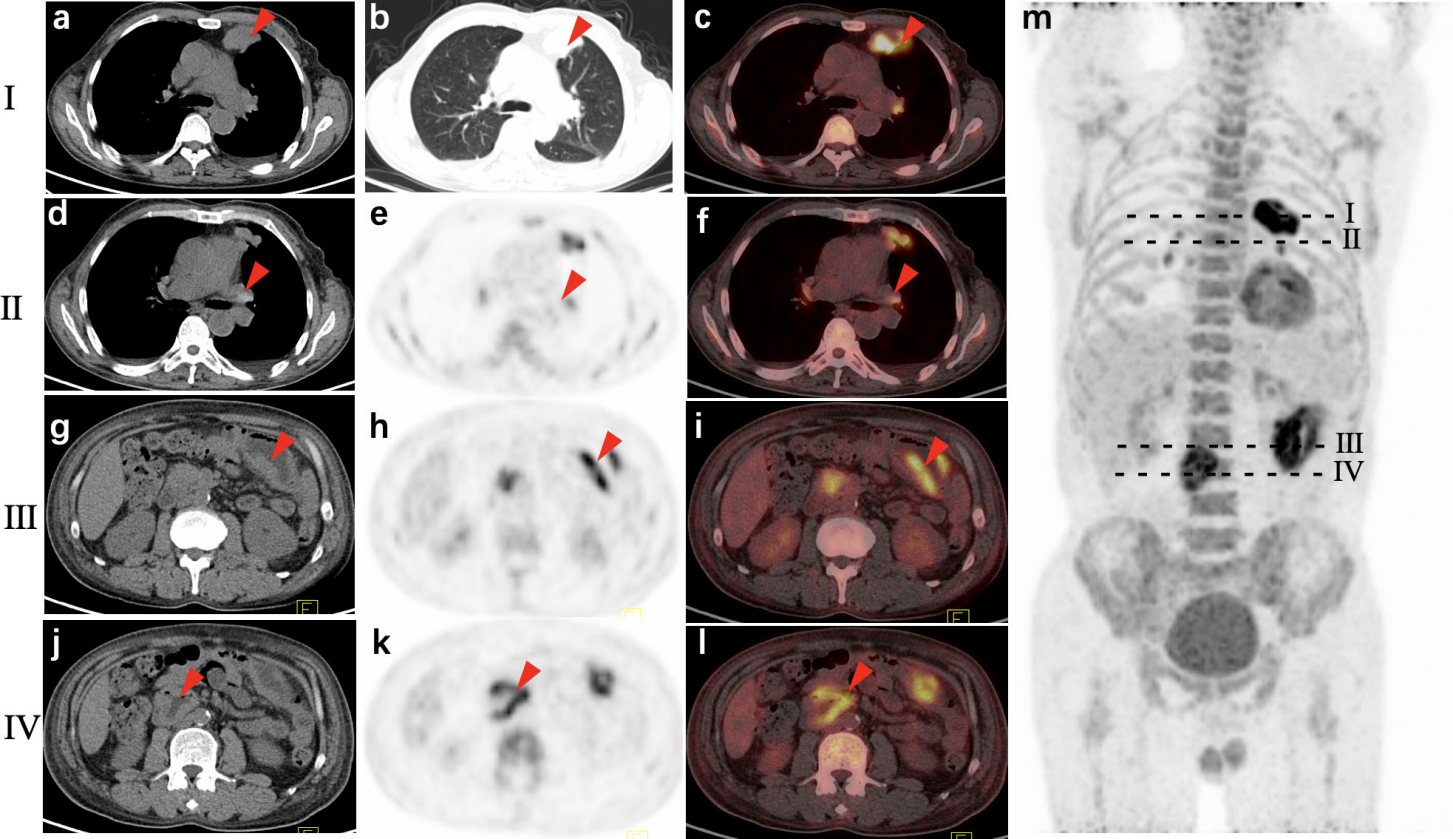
^18^F‐FDG PET/CT image of Case. I. (a) Soft tissue window; (b) lung window; and (c) PET/CT fusion image of left lung lesion manifested an irregular left upper lobe lung mass measuring 4.7 × 3.3 × 3.5 cm, with SUVmax of 13.4. The lesion showed malignant features including irregular margin, lobulation, spiculation, pleural traction, obvious necrosis of more than 30%, but without bronchial stricture and occlusion. ^18^F‐FDG PET/CT also detected wall‐thickening of descendant duodenum and part of jejunum in left upper quadrant of the peritoneal cavity with SUVmax of 10.4. The local intestinal cavity was dilated, and the surrounding fat gap was clear. II. (d) Transaxial CT; (e) PET; and (f) PET/CT fusion of bilateral hilar lymph nodes mildly enlarged with high density and symmetrically increased ^18^F‐FDG uptake (SUVmax: 4.0–5.3). III and IV. (g) Transaxial CT; (h) PET; (i) PET/CT fusion image of jejunum lesion; (j) transaxial CT; (k) PET; and (l) PET/CT fusion image of duodenum demonstrated thickening of the intestinal wall with intensive FDG uptake and dilatation of local intestinal cavity. (m) Maximum intensity projection image of whole body showed high uptake in lesions (red arrow) in left thorax (I); hilar lymph node (II); left middle upper abdomen (III); and right middle abdomen (IV).
